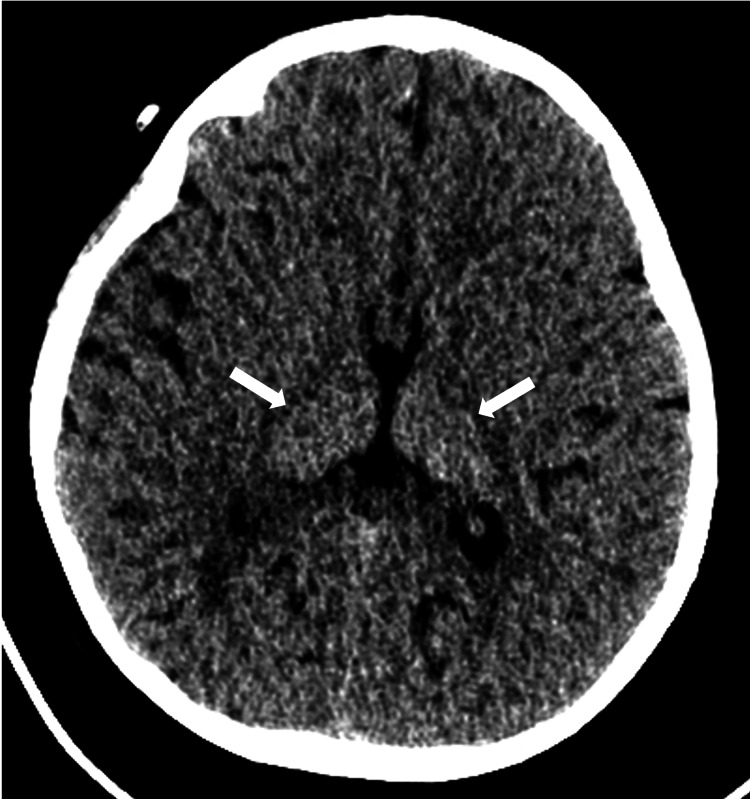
Axial CT Axial non-contrast enhanced CT image in stroke window setting, at the level of basal ganglia obtained at 5 years of age showed relative thalamic hyperattenuation (white arrows).
